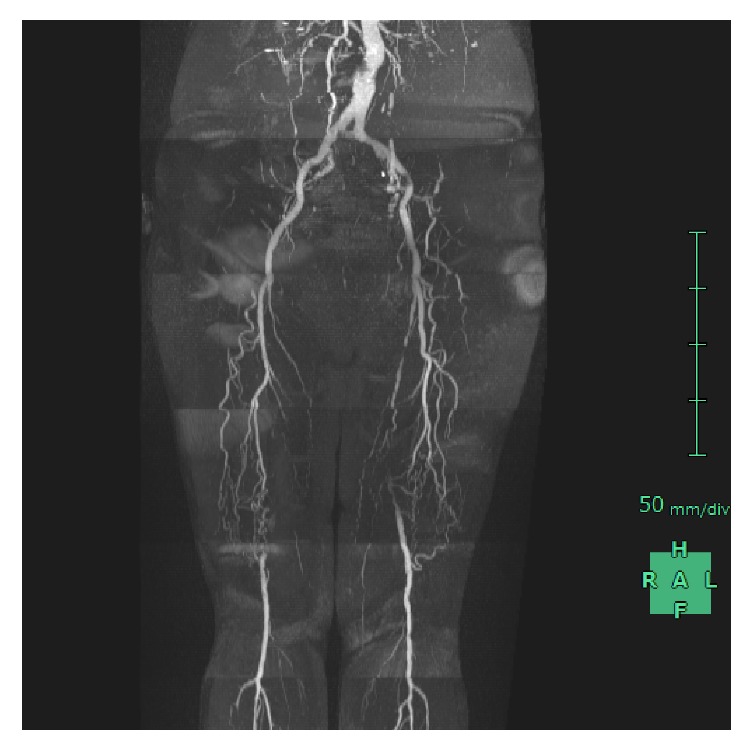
MRI showed that both of the patient's SFAs were already occluded from bifurcation of the deep femoral artery. MRI: magnet resonance imaging; SFA: superficial femoral artery.
